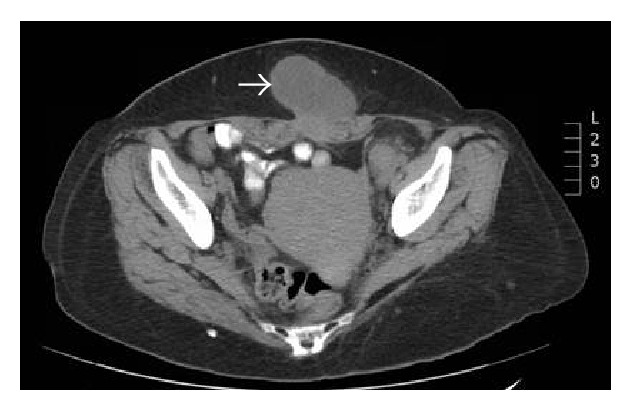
Abdomen and pelvis computed tomography (CT) scan revealed a 6.0-centimeter mass (arrow) involving linea alba, bilateral rectus abdominis, and subcutaneous fat. The mass was not attached to any organs. Also noted was prominent left external iliac and inguinal lymphadenopathy.
